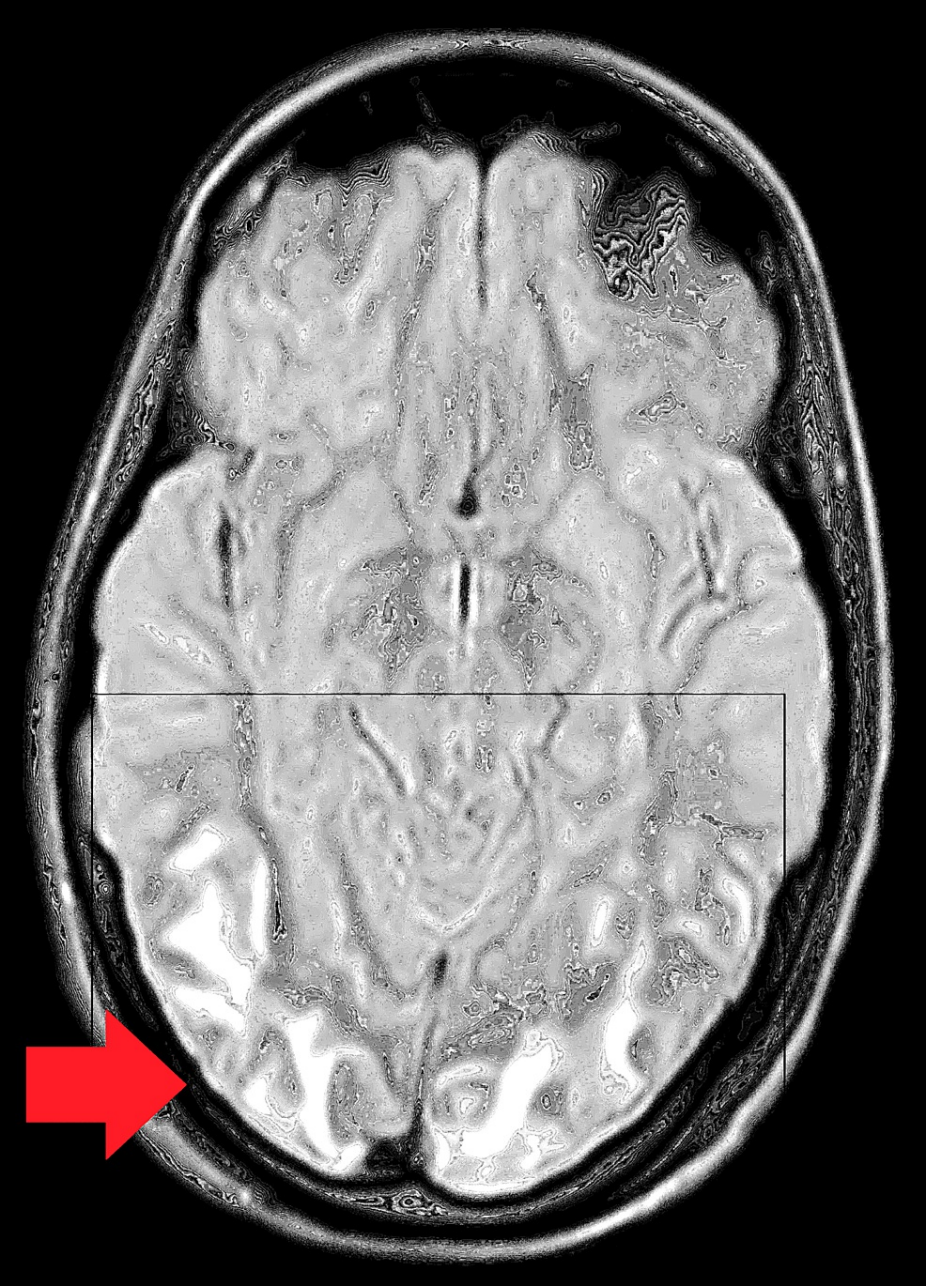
Abnormal hyperintense lesion confluent in both parieto-occipital lobes upon FLAIR scan. FLAIR: Fluid-Attenuated Inversion Recovery.
